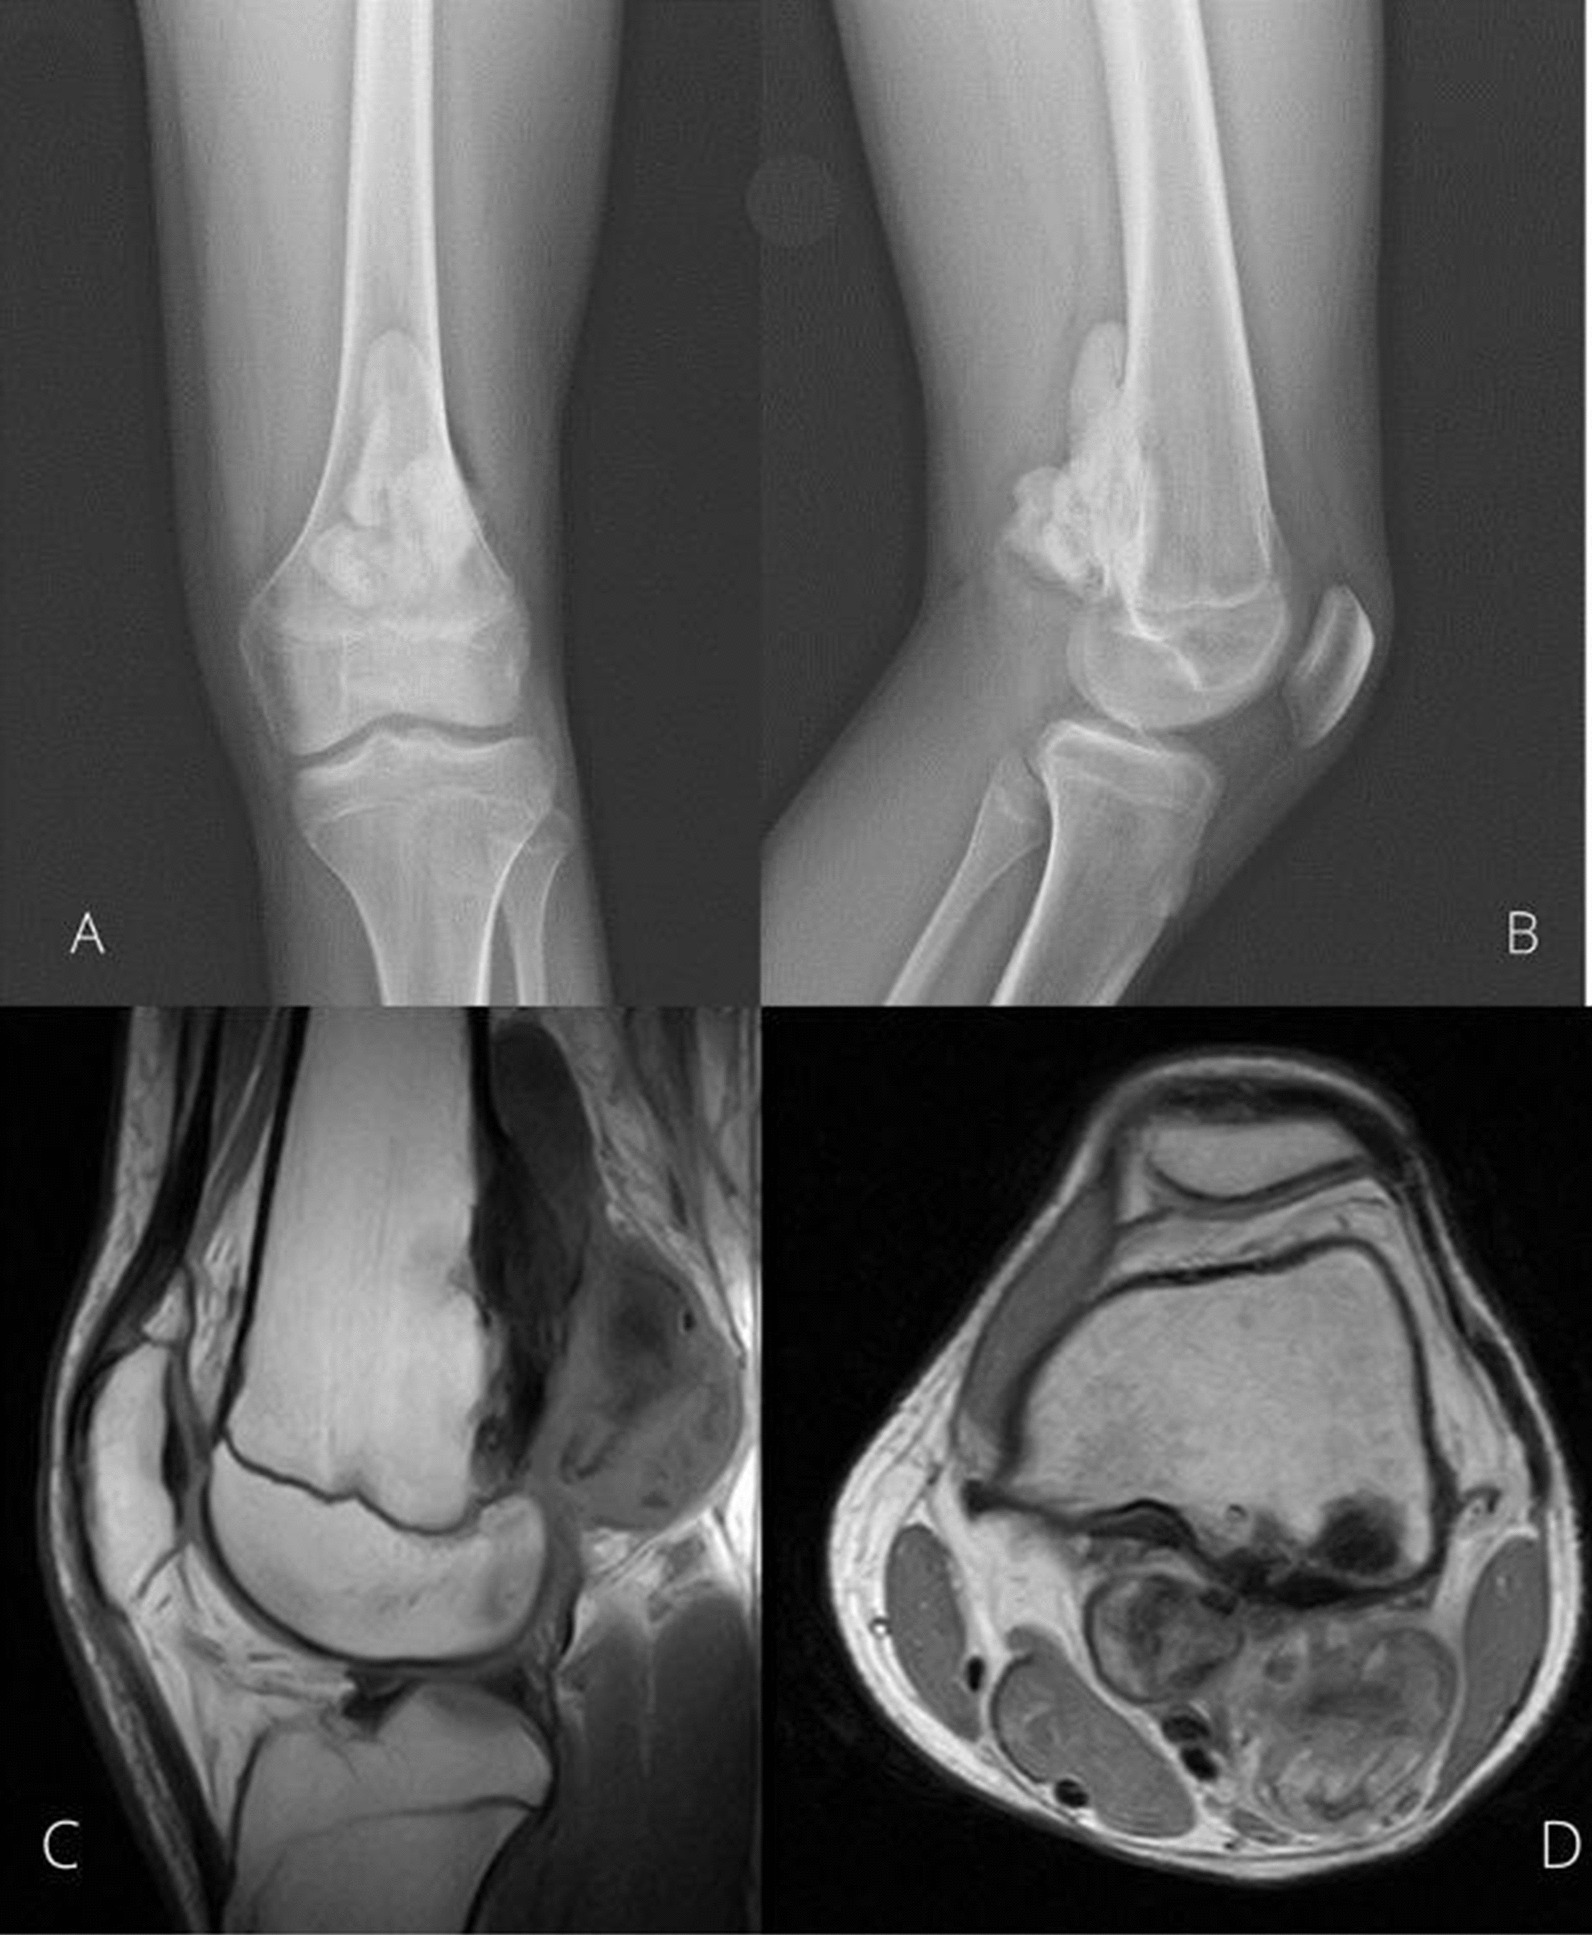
A, B Antero-Posterior and Lateral X-rays of the left knee show a lobulated, radiodense lesion protruding over the posterior cortex of the distal femur. It attaches to the underlying cortex with no invasion into the medullary bone. C, D: MRI: sagittal and axial scan of a T1-weighted image of the left distal femur of the patient. A protruding mass, with low signal intensity, arising from the posterior cortical of the femur can be seen. The medullary canal and the cruciate ligaments are not involved
